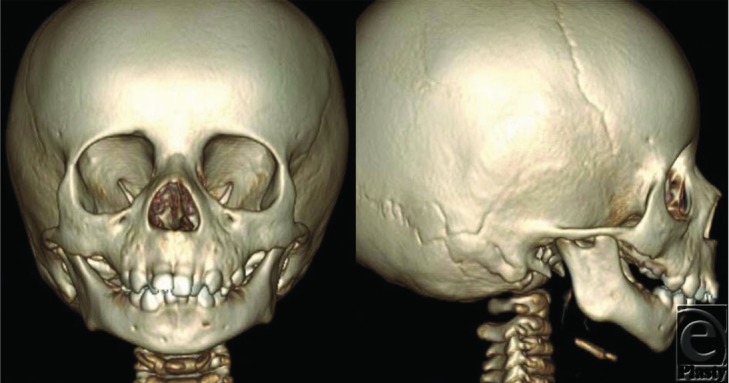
Preoperative 3-dimensional reconstructed maxillofacial computed tomographic scan.
